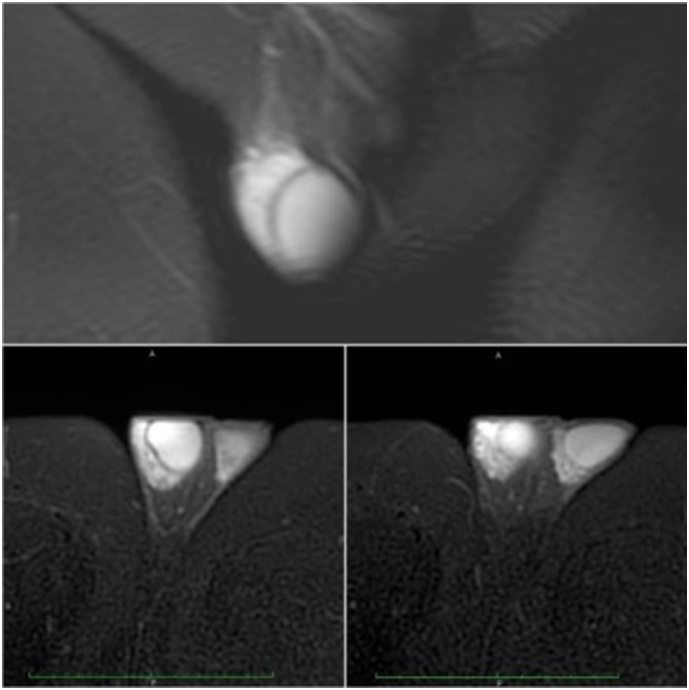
MRI. MRI after completion of antibiotic therapy for further evaluation of the proposed diagnosis in acute phase by sonography; In axial and coronal T2 FISP images, hemitesticular structure with similar signal intensity to that of the adjacent normal right testis is seen, now decreased in size due to resolution of inflammatory phase.
